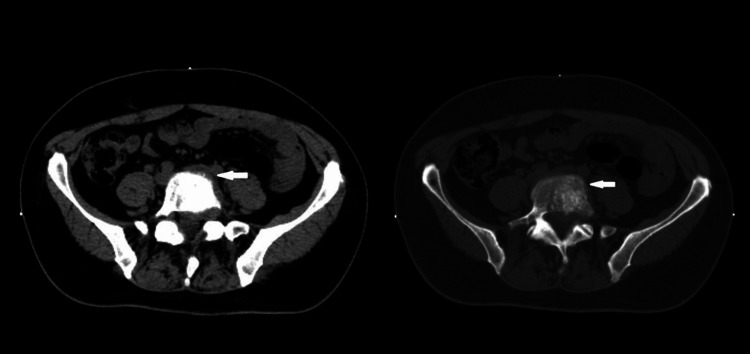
Lumbar spine CT scan, axial plane. A lumbar spine CT showed a lesion involving the body, left pedicle, and left superior articular facet of the L5 vertebrae.
